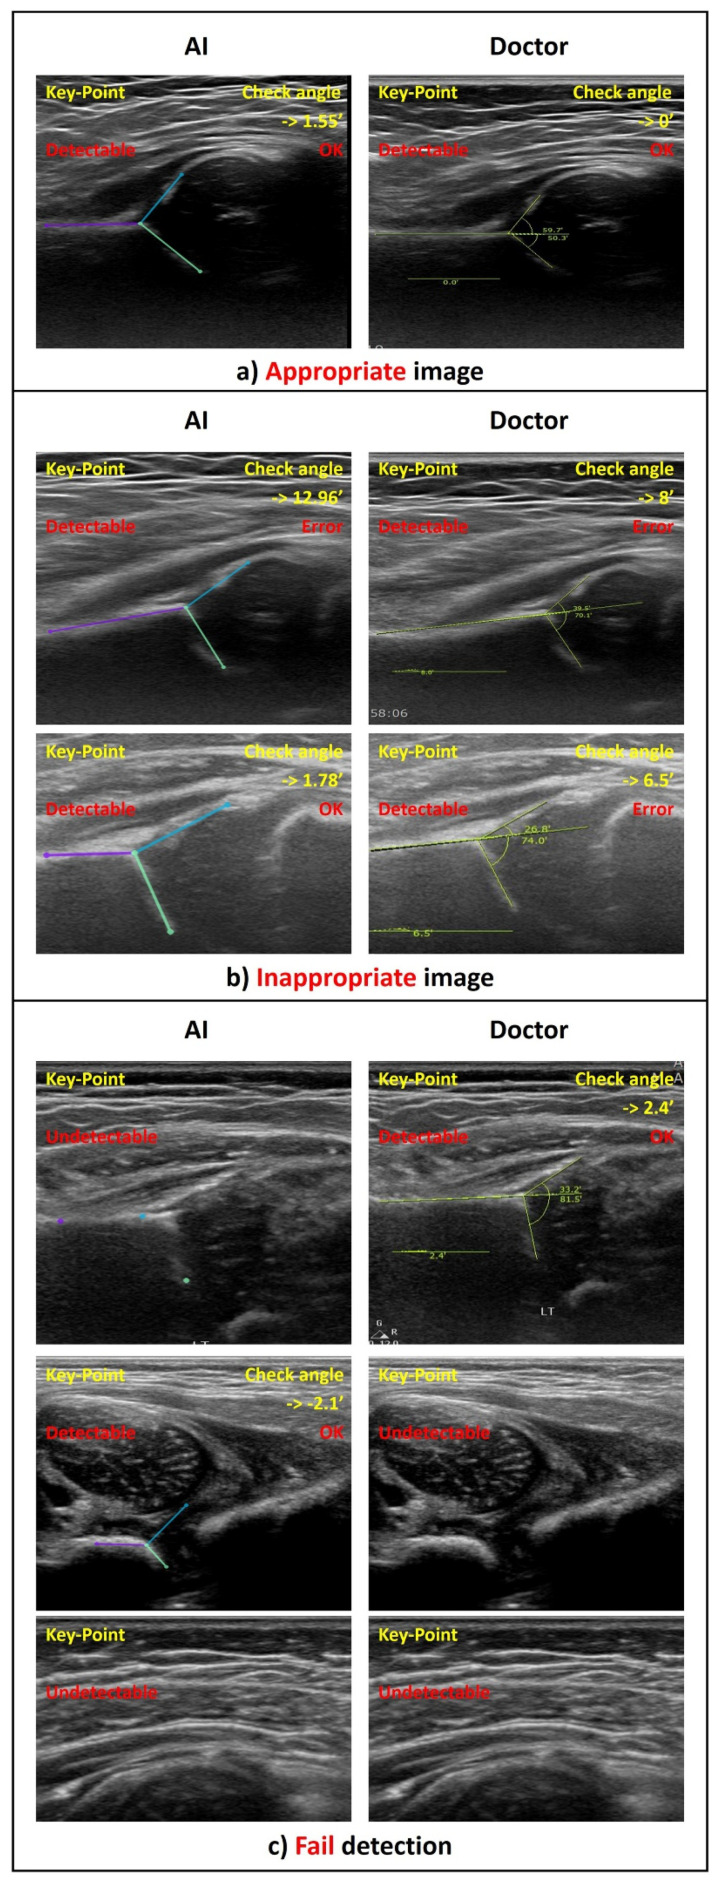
Examples of ultrasonographic image classifications in the study: (a) Case of the appropriate image where detection of all key points was possible, the measured check angle was less than 5° for both observers, and the alpha and beta angles were evaluated (*n* = 320). (b) Case of the inappropriate image where detection of all key points was possible, but the check angle was more than 5° for at least one observer (*n* = 192). (c) Case of the fail detection where at least one observer could not detect all key points (*n* = 409).
